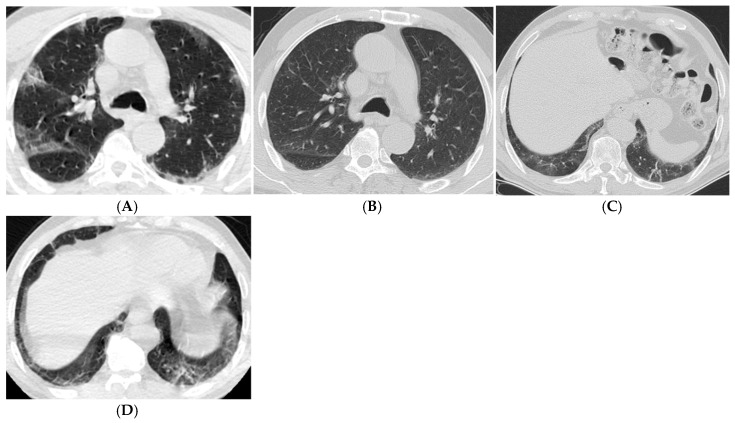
Axial CT scans of a 67-year-old male patient with moderate COVID-19. (A,B) The CT obtained 9 days after the onset of symptoms shows peripheral ground-glass opacities, consolidation areas, and curvilinear and linear fibrotic bands on both sides, with a CT severity score of 16. (C) The follow-up CT performed two years later reveals no significant pathological findings at the carinal level compared to the previous examination. (D) In the left lower lobe, more pronounced patchy, blurry ground-glass densities, thick parenchymal bands, and persistent fibrotic densities are observed in the posterior basal segments.
